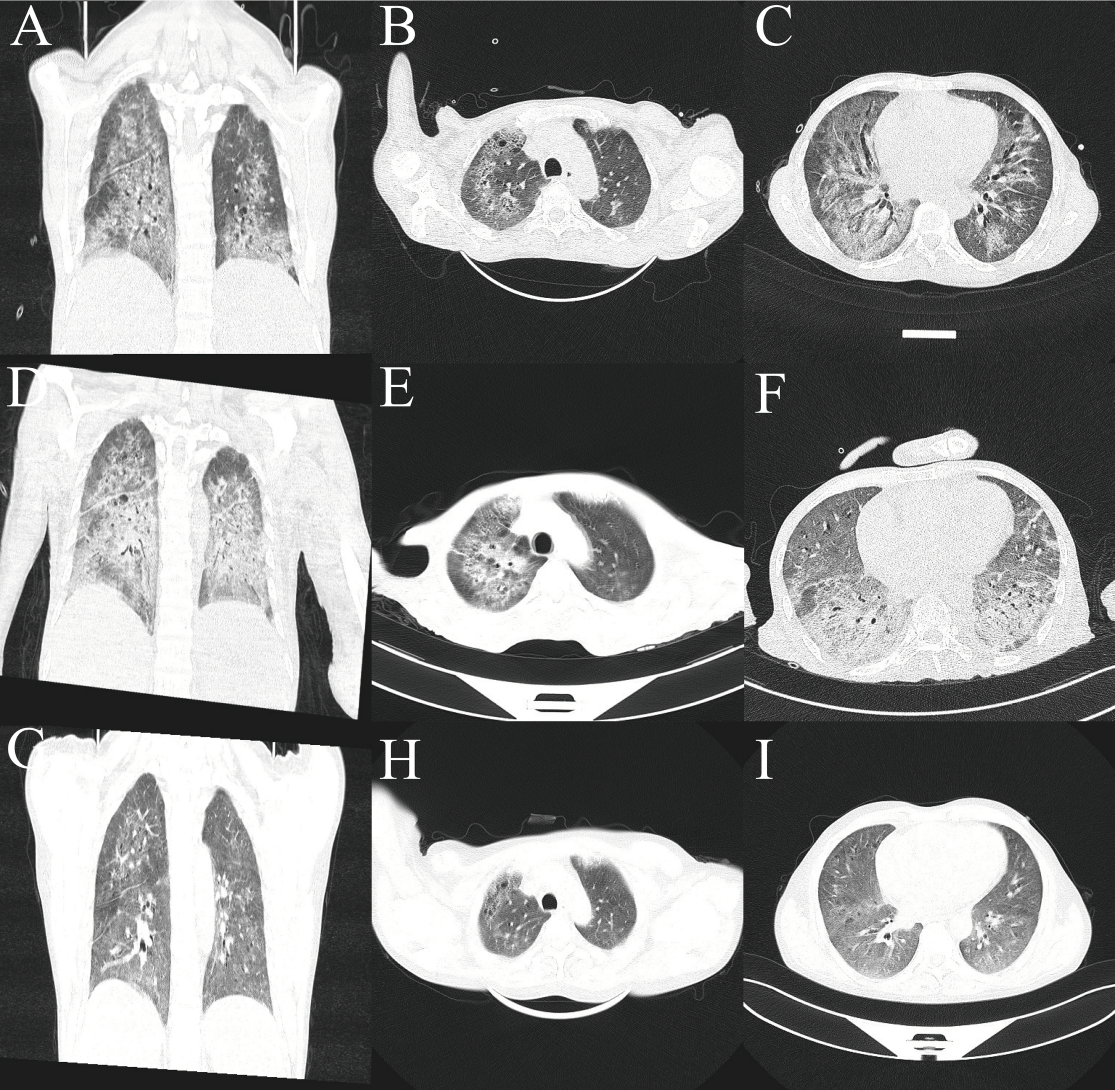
Lung computed tomography. (A–C) At admission. (D–F) Axial view after methylprednisolone and cyclophosphamide therapy. (G–I) After treatment using ruxolitinib, a *JAK2* inhibitor.
